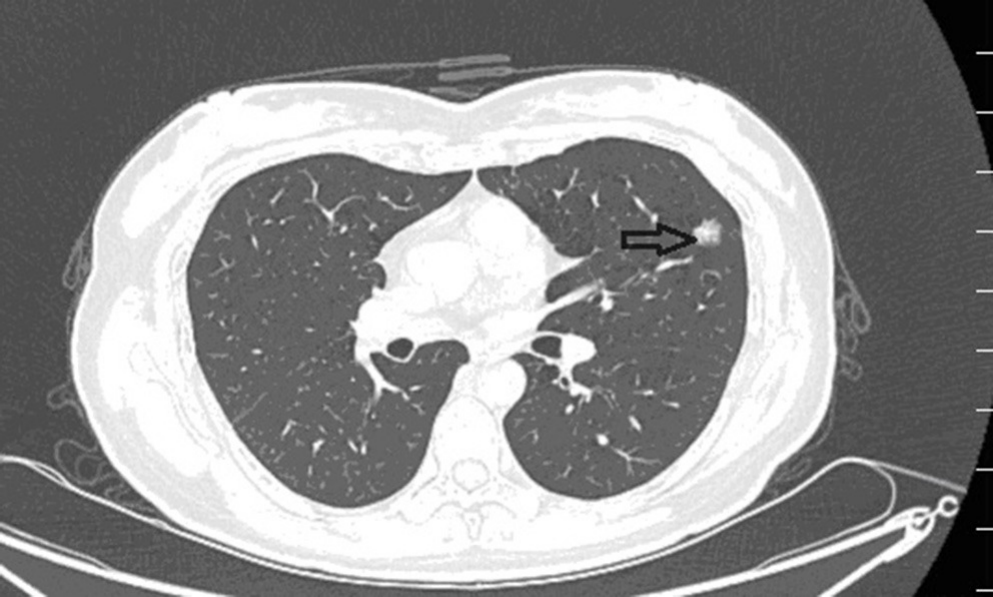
Preoperative contrast-enhanced CT images revealed a ball-shaped mass with heterogeneous enhancement in the left lung lobe.
